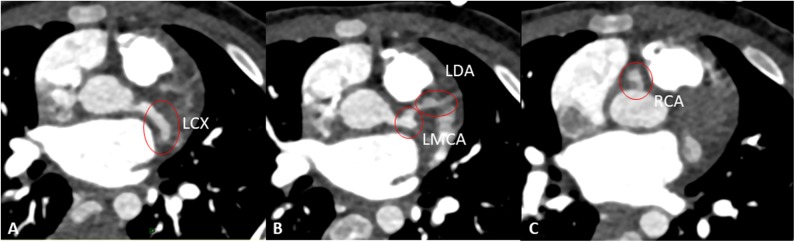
Angio-CT showing aneurisms of the left circumflex artery (LCX, A), left main coronary artery (LMCA), and left anterior descending artery (LAD) (B), and right coronary artery (RCA) (C).
